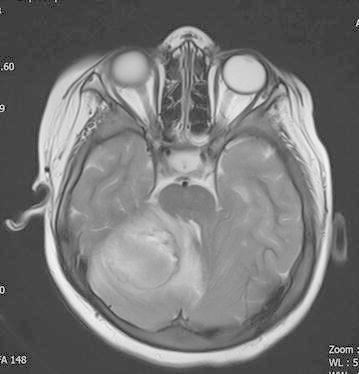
Preoperative cranial MRI showed a 39 × 34 mm mass lesion
